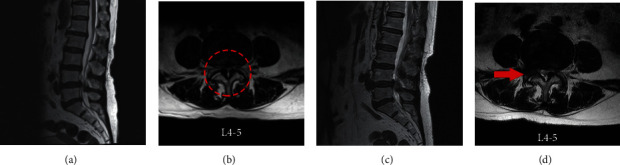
MRI scans of a case with lumbar stenosis at L4-5 with “U” route PELD. (a, b) Preoperative sagittal and cross-sectional MRI images, the lumbar stenosis, and disc herniation are indicated by the red circle. (c, d) Postoperative MRI images are showing ventral and dorsal decompression. The red arrow indicates that the removal of partly ventral osteophyte of SAP, herniated NP, and hypertrophic LF at L5. SAP = superior articular process, NP = nucleus pulposus, and LF = ligamentum flavum.
